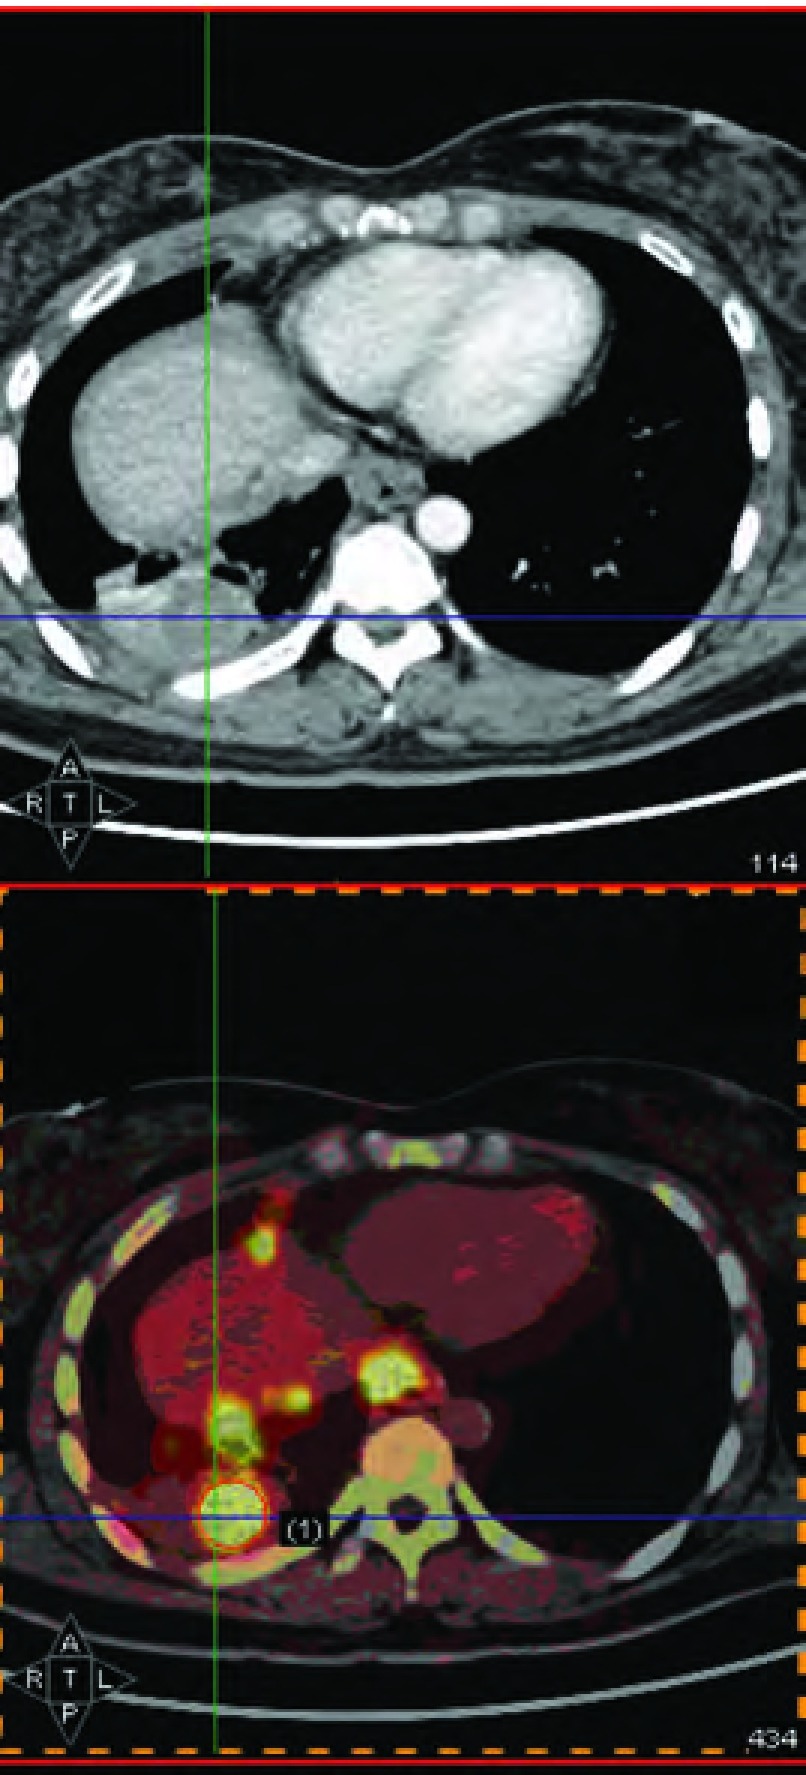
PET/CT显示右侧胸腔积液，胸膜增厚，SUVmax升高，诊断肺癌合并恶性胸腔积液。 PET/CT shows right-side pleural effusion and reveals nodular thickening increased uptake in parietal pleura.
